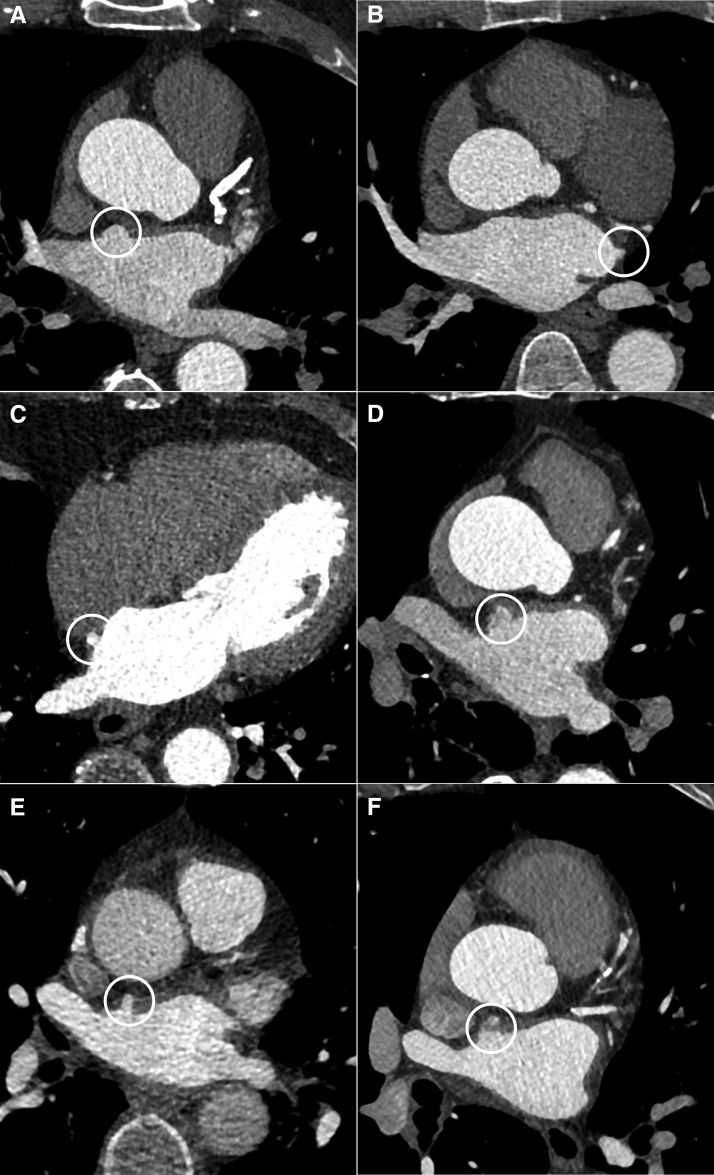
Axial images of acute cardiac computed tomography portraying left atrial diverticula. A, Right superior location with a cystic shape, (B) left superior location with a cystic shape, (C) right inferior location with cystic shape, (D) trabeculated endocardial surface (E) tubular shape (F) right superior location with hypodensity within outpouching, suggesting slow-flow (Hounsfield units >100).
